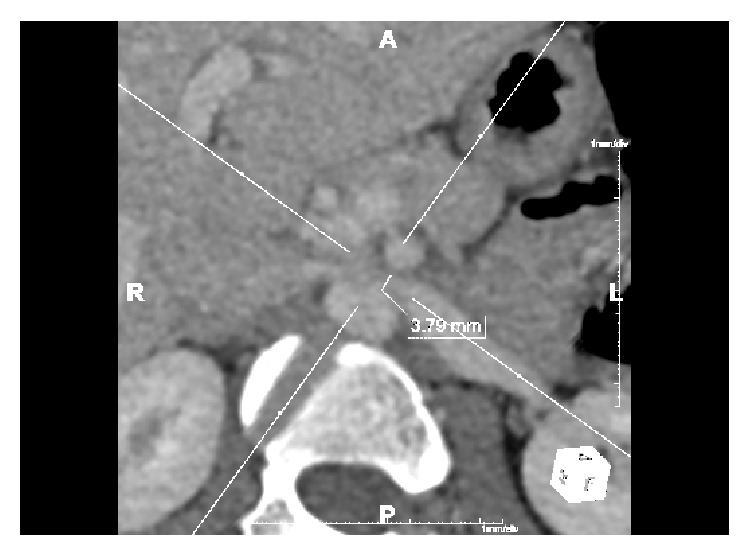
Axial contrast CT in our patient showing a LRV diameter of 3.79 mm due to mesoaortic compression. A reduction in diameter of ≥50%.
